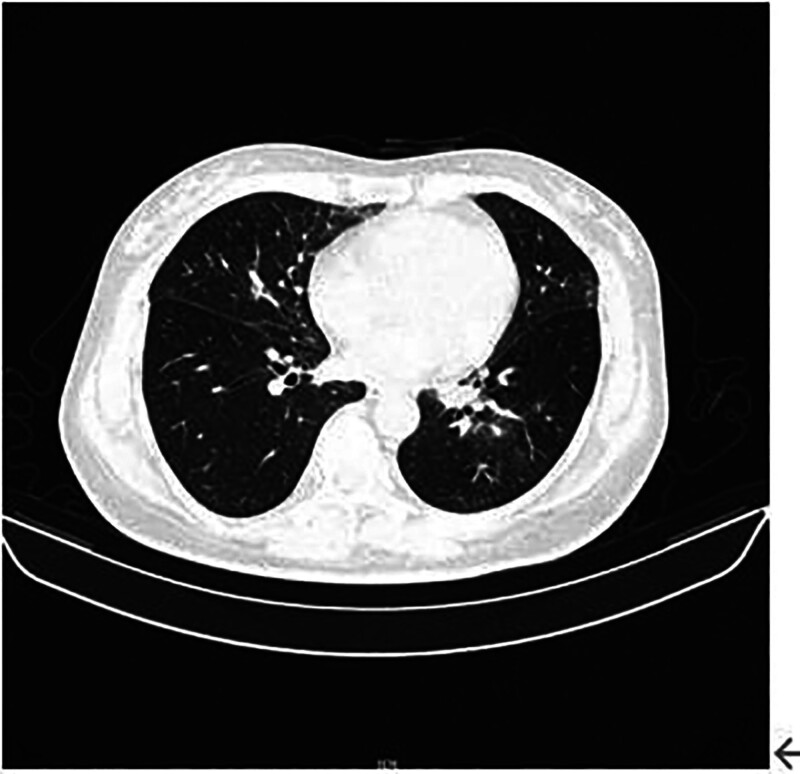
Patient was reexamined using pulmonary CT on October 18, 2023. CT = computed tomography.
